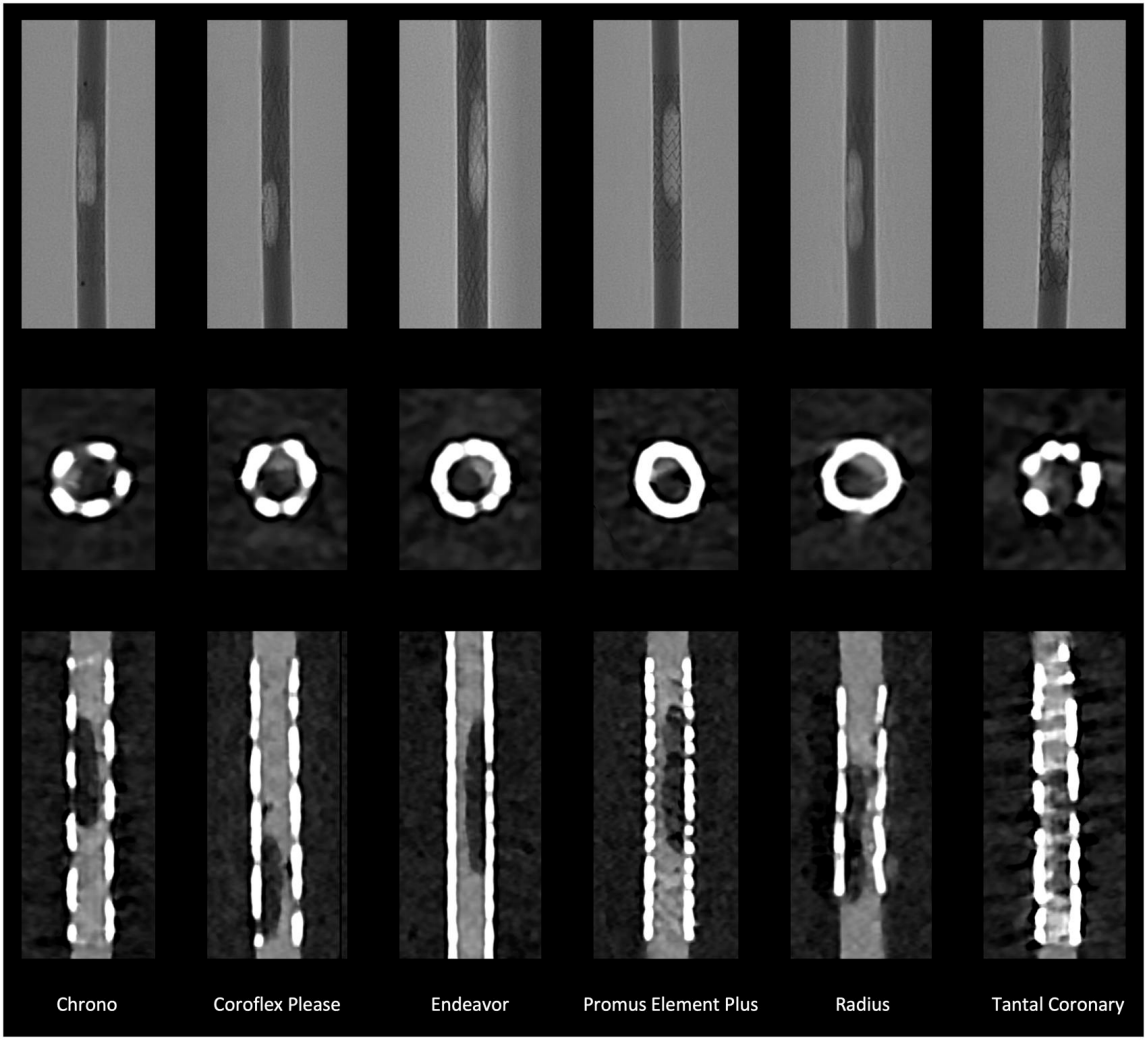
Angiography and PCCT of in-stent stenosis. The first row shows the angiographic image of the stents with the stenoses in optimal projection for each stent used. The second row shows the axial reconstruction, the third the corresponding longitudinal reconstruction of the CCTA. CT images reconstructed with UHR data sets and the optimized Bv72 kernel. Abbreviations: PCCT = photon counting CT; UHR = ultra-high-resolution mode.
